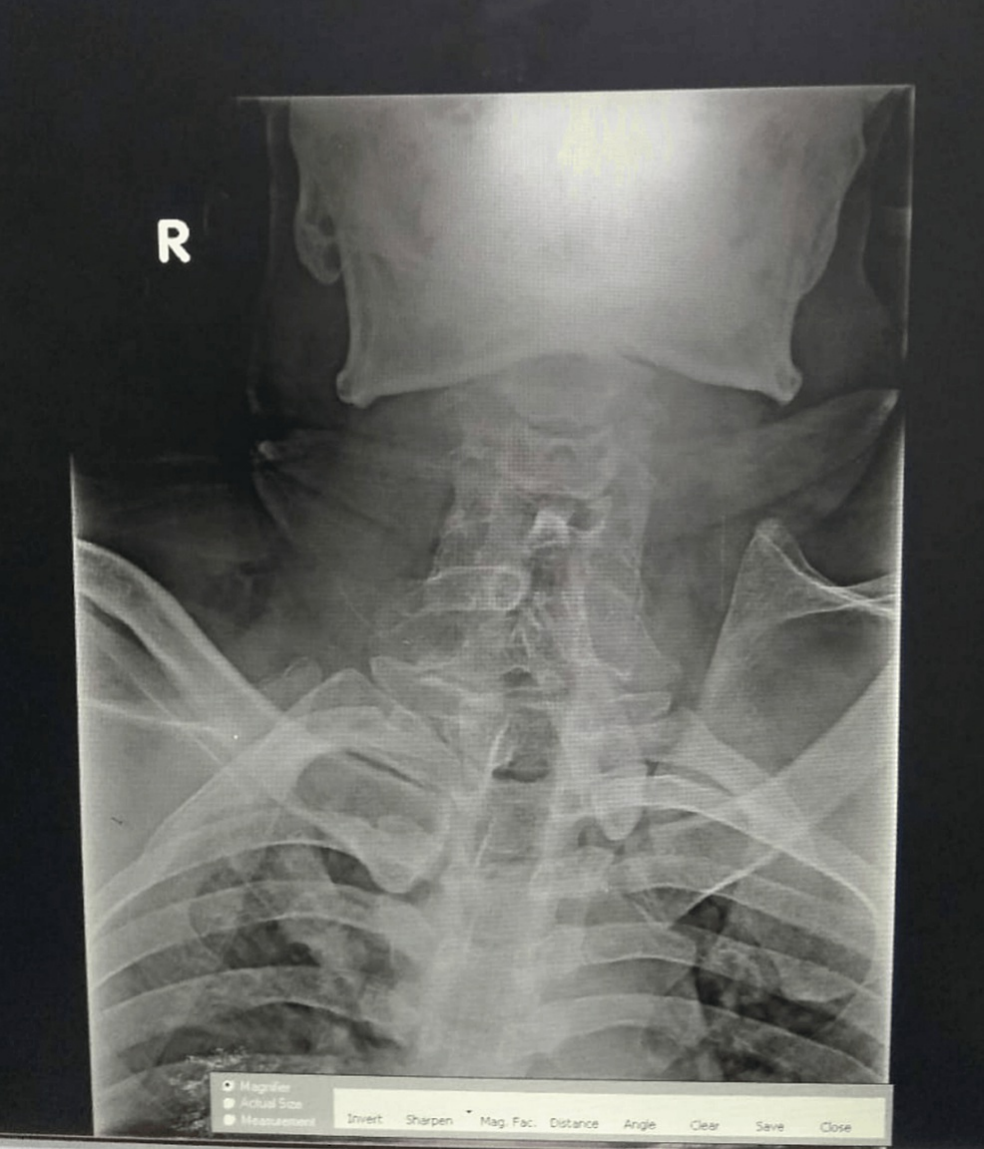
X-ray of the neck showing the fused cervical vertebra and large spinous process
